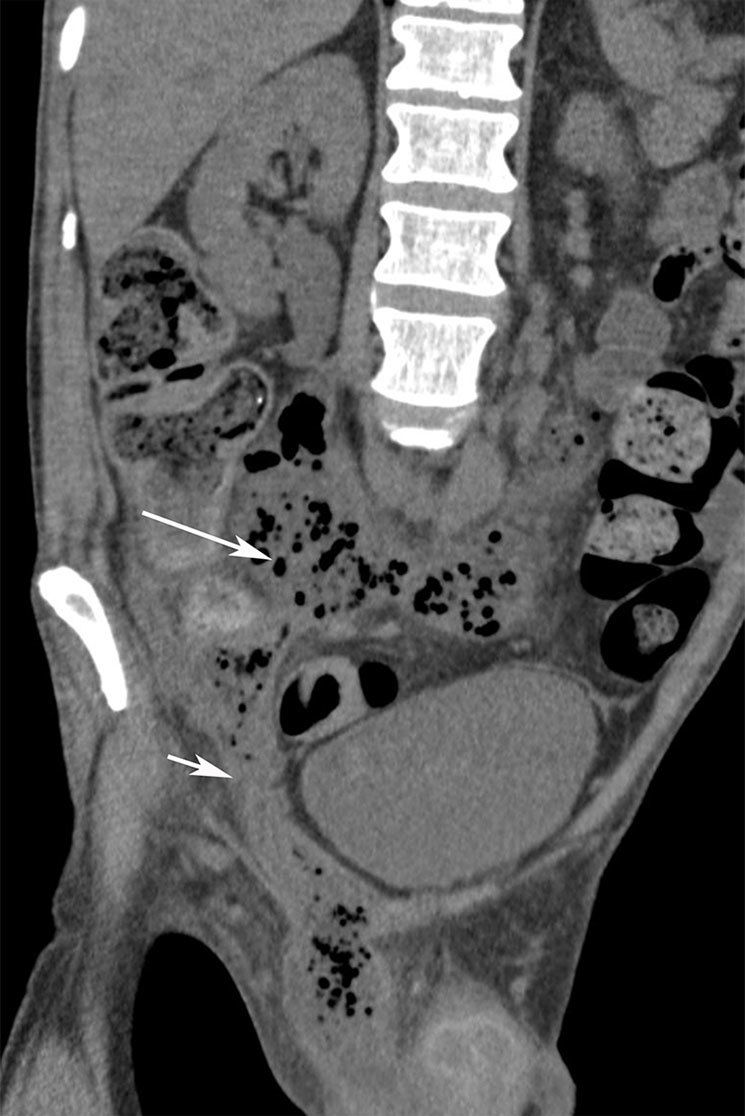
Coronal oblique venous phase contrast-enhanced CT: long arrow points to a large retroperitoneal abscess while the short arrow points to the tracking *via* the obturator space into the scrotum.
